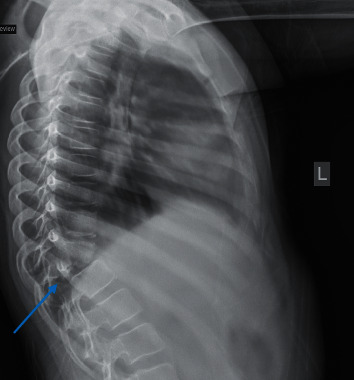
Lateral spine X-ray demonstrates destruction of the thoracic vertebra (T11) body and inferior end plate of the T10 vertebra with loss of disk spaces between T10, T11, and T12.
